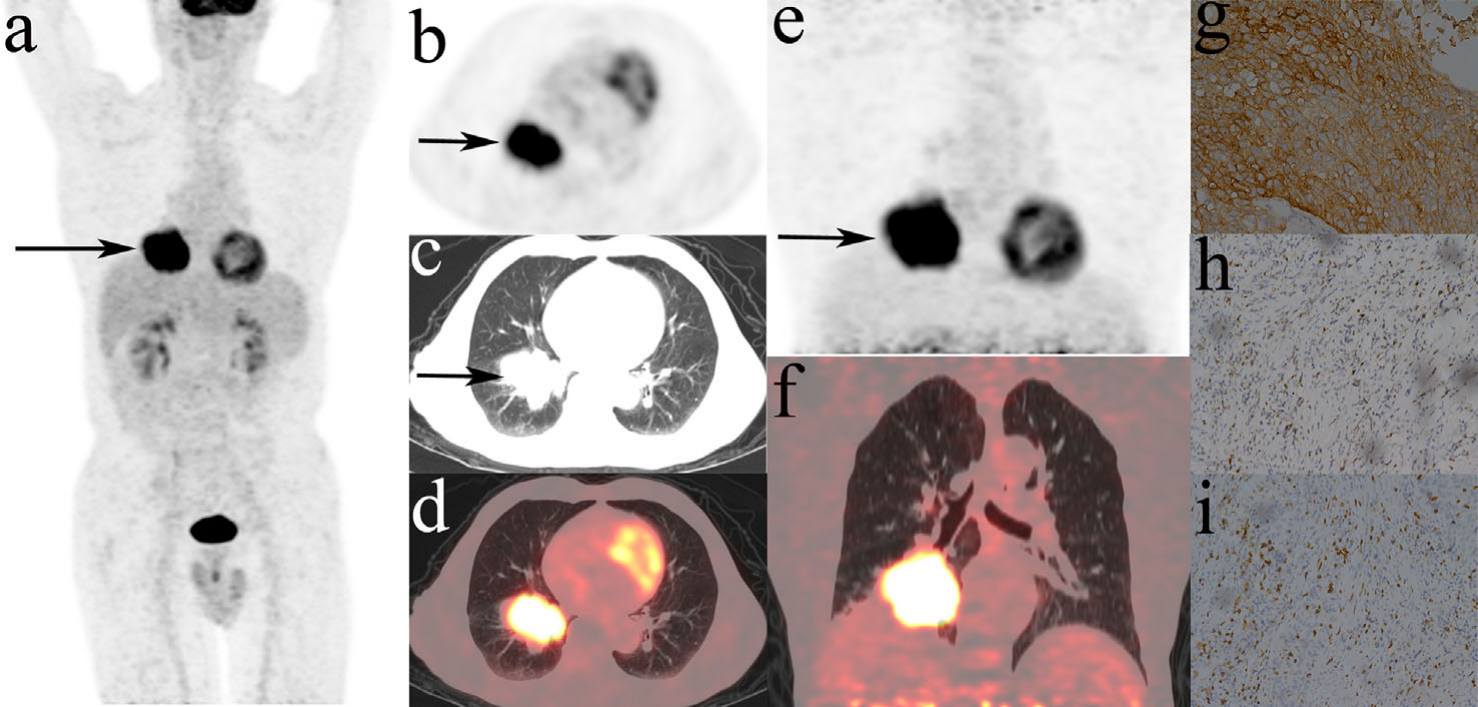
Representative DTP ^18^F-FDG PET/CT imagings of a 71y male patients, defined as TMIT I tumor. (A–D): early images, (E, F): delayed images, (A): MIP figure, (B–D): PET, lung window, PET/CT fusion image. A mass was in the lower lobe of right lung (arrow) with markedly increased radioactivity, eSUVmax: 20.3, eSUVmean: 12.0, eMTV: 52.9 cm^3^, eTLG: 634.8 g, dSUVmax: 27.7, dSUVmean: 16.0, dMTV: 49.16 cm^3^, dTLG: 786.56 g. The surgical pathology: moderately differentiated squamous cell carcinoma. (G) high PD-L1 expression. (H) PD-1 TIL high density. (I) CD8^+^ TIL high density.
